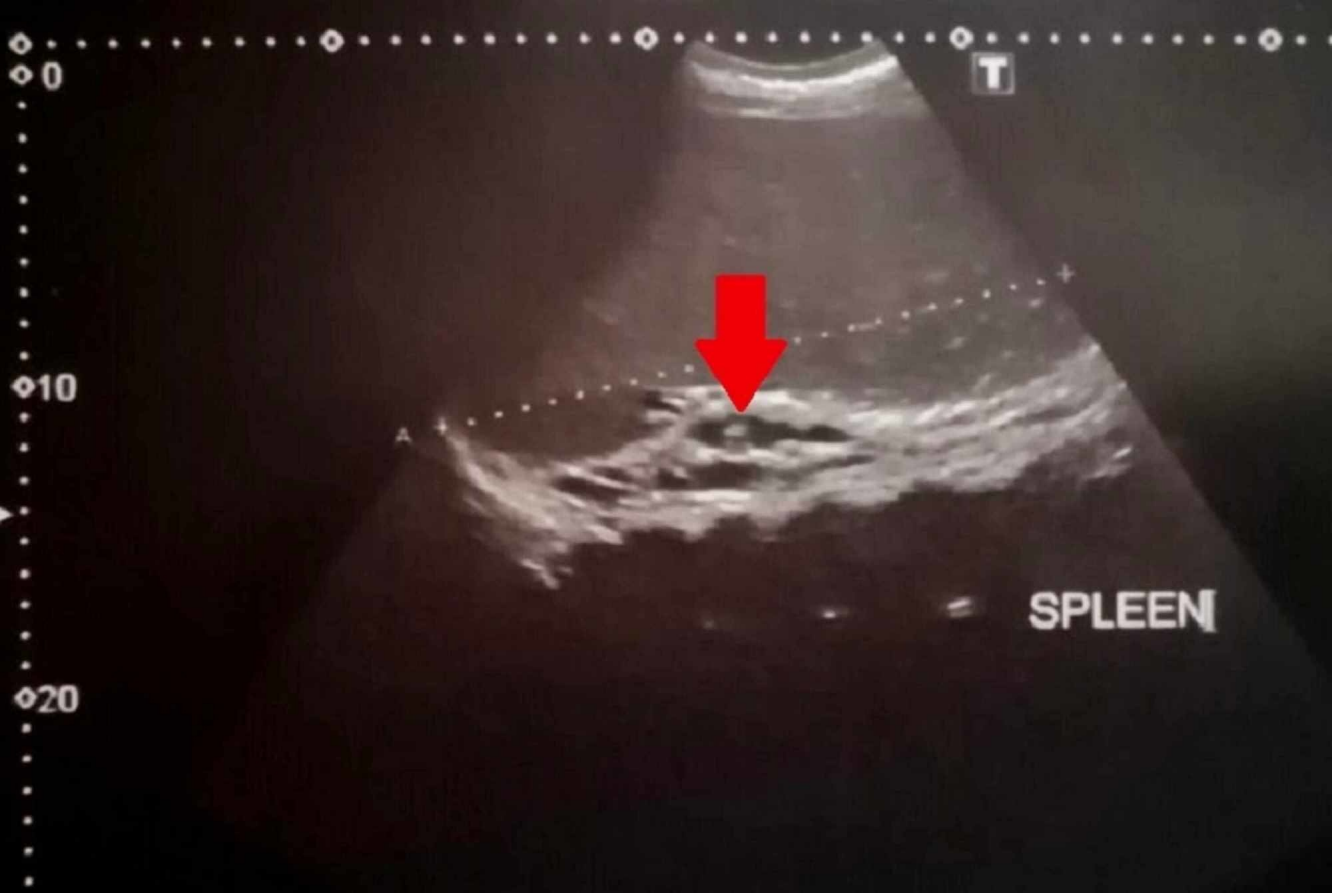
Doppler US showing dilated varices at splenic hilum (red arrow) US, ultrasound
